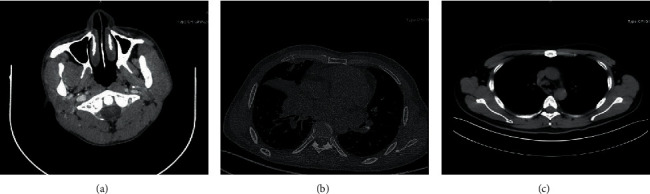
Parotid gland CT scan revealed an enlarged, substantially inhomogeneous left parotid gland with an unclear border mass (a). Chest CT scan revealed a lobulated soft mass with a vague margin located in the right lung middle lobe accompanied by stenosis of the local lumen leading to atelectasis of the right middle lobe (b). Chest CT scan also showed an irregularly shaped mass located in the bifurcation of the trachea (c).
